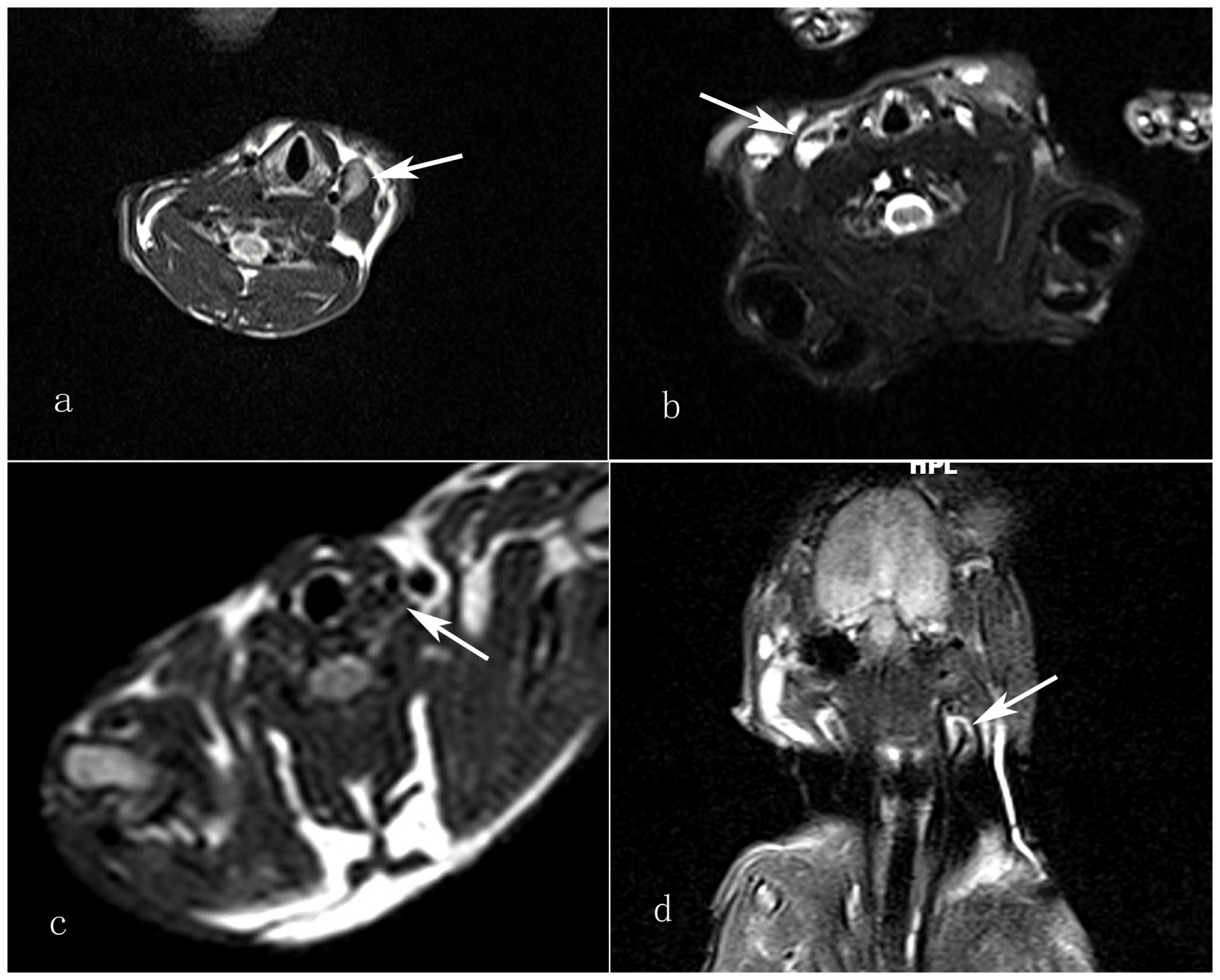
T2-weighted image of the neck shows a metastatic lymph node with partial high signal intensity, significantly higher than that of muscle tissue. (white arrow) b. T2-weighted image of the neck shows the metastatic lymph nodes that had irregular signal reduced intensity (white arrow). c. The inflammatory lymph nodes showed homogeneous signal falls on T2-weighted image. (white arrow) d. The metastatic lymph nodes also had irregular signal reduced intensity on coronal view (white arrow).
